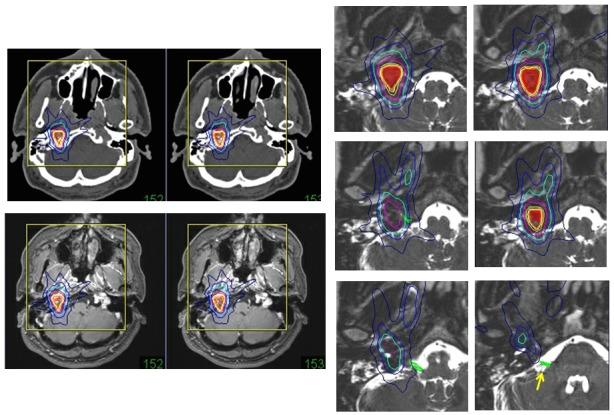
A jugular foramen tumor and the surrounding structures. A Gd-enhanced MR image (lower left) fused to thin-sliced CT (upper left). The image fusion of a heavy T2-image showing the dose plan sparing the 9th-11th nerves (green) and 8th nerve (green with a yellow arrow).
